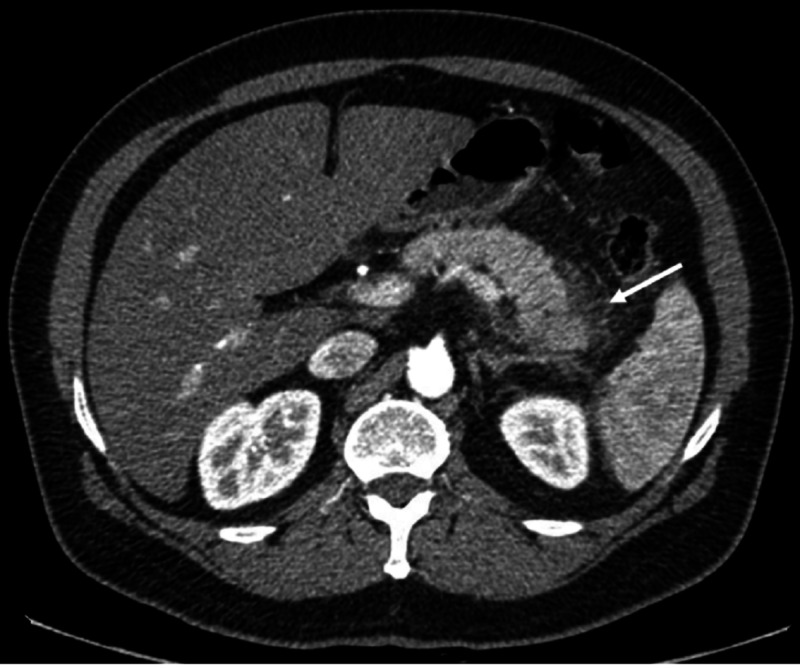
CT abdomen with contrast on day 3 CT scan showing features of pancreatitis and peripancreatic inflammation (arrow)
